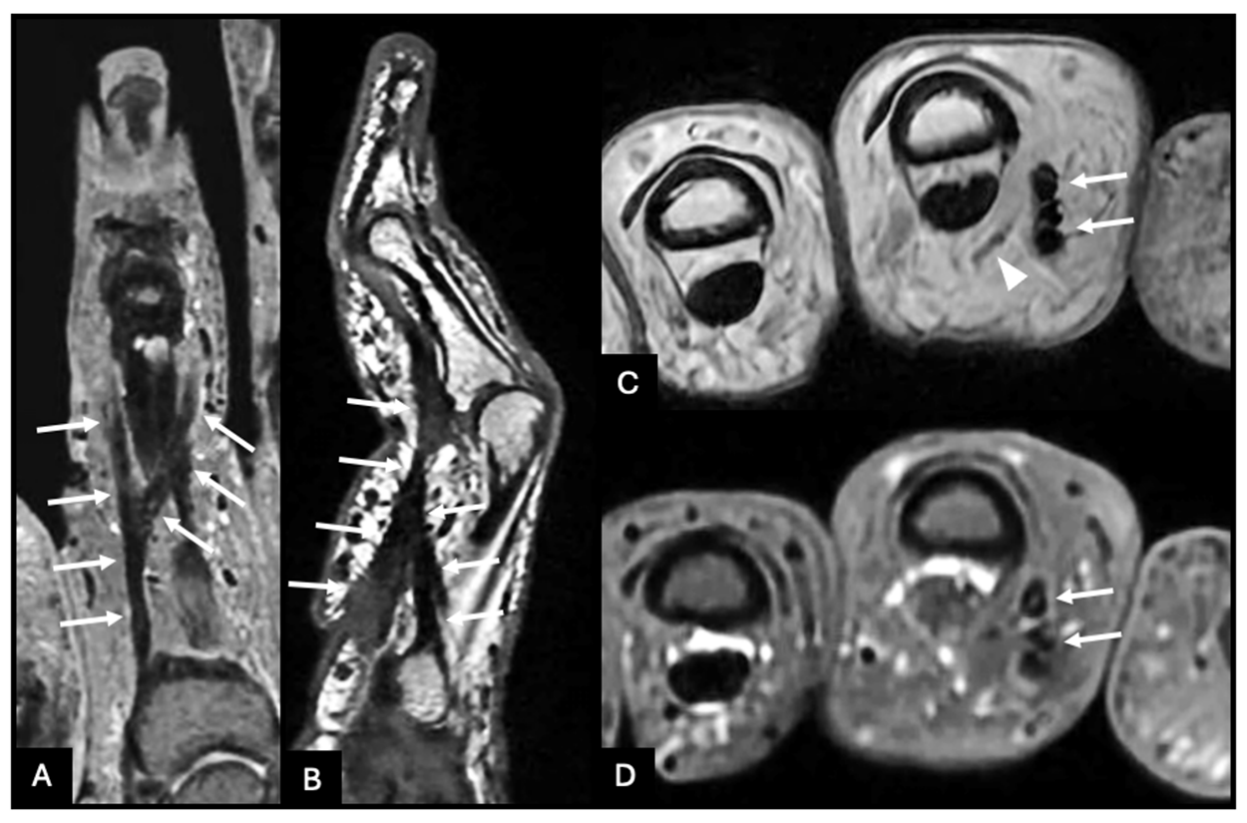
Magnetic resonance imaging showing a hypodermal fibrous cord along the radial and palmar aspects of the proximal phalanx (arrows), bifurcating distally into two slips inserting on the base of the middle phalanx. The radial neurovascular bundle is displaced in a characteristic pattern without encasement (arrowhead).
